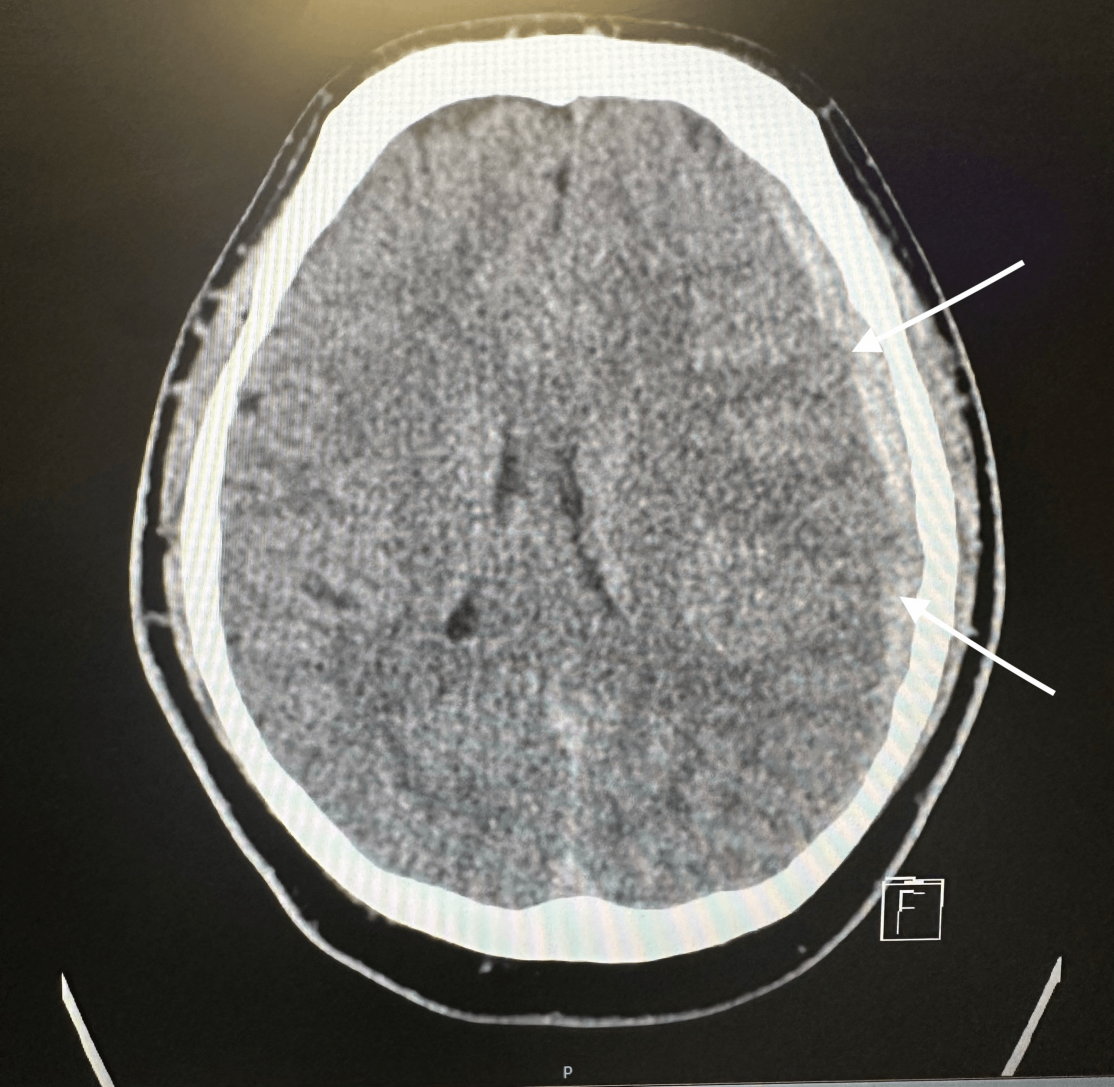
Transverse CT image prior to craniotomy demonstrating SDH CT, computed tomography; SDH, subdural hematoma
